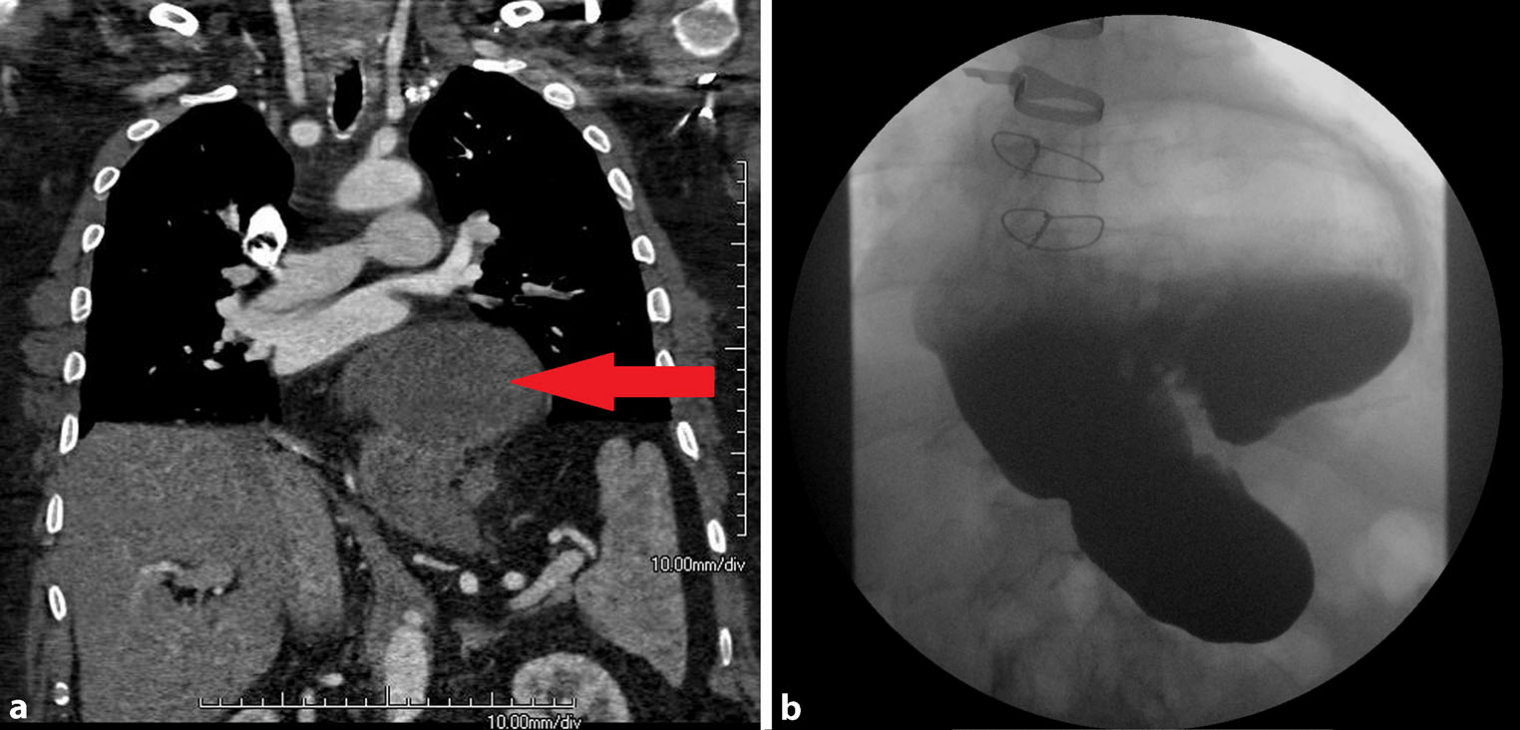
Computed tomography (a) and gastrointestinal contrast series (b) show a mixed axial and para-oesophageal upside-down stomach (*red arrow*) compressing the left ventricle, without any incarcerated portions of the stomach
